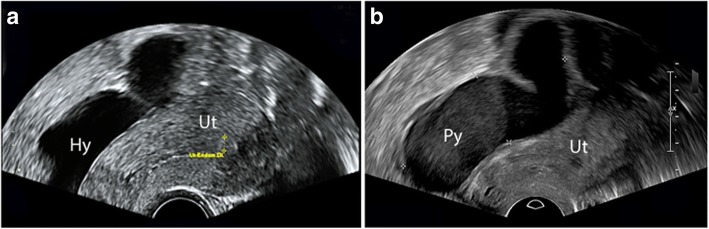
a Transvaginal ultrasound performed 3 months prior to patient admission during a routine gynecological visit, showing a tubular structure of 8.1 × 2.0 cm in size filled with anechoic fluid on the left side of the uterus and interpreted to be a hydrosalpinx. b Transvaginal ultrasound at admission, showing a tender tubular structure of 7.3 × 2.1 cm in size filled with low-level echogenic fluid in the left pelvis and interpreted to be a pyosalpinx. *Ut = uterus; Hy = hydrosalpinx; Py = pyosalpinx*
